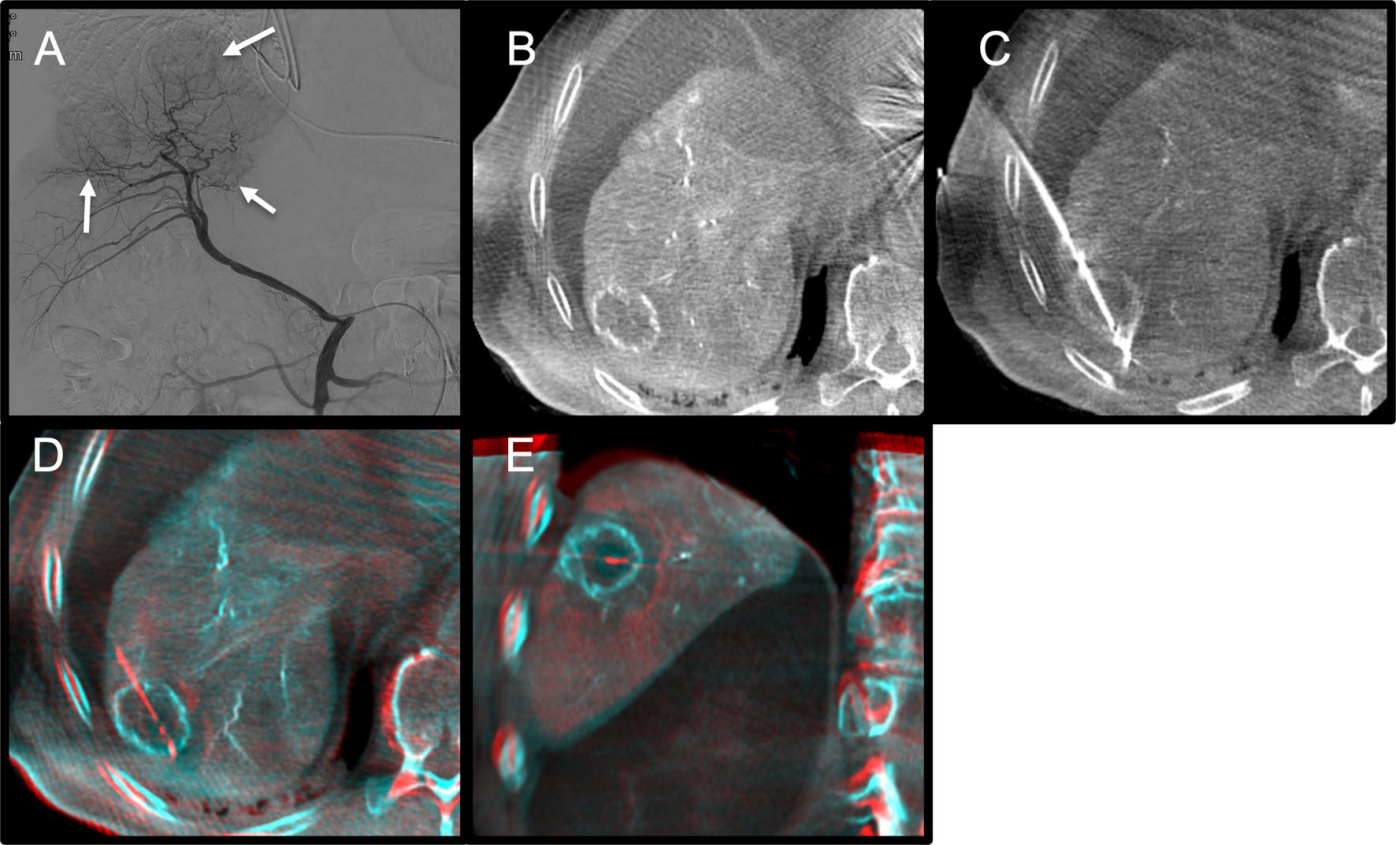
Example of a hypervascular hepatocellular carcinoma (HCC) ablation. A. Digital subtraction angiography of the right hepatic artery shows three hypervascular HCCs. B. C-arm CT with hepatic arteriography shows the first target lesion. C. Antenna in position. D and E. Fused pre- and post-ablation C-arm CTs depicting ablation margins
